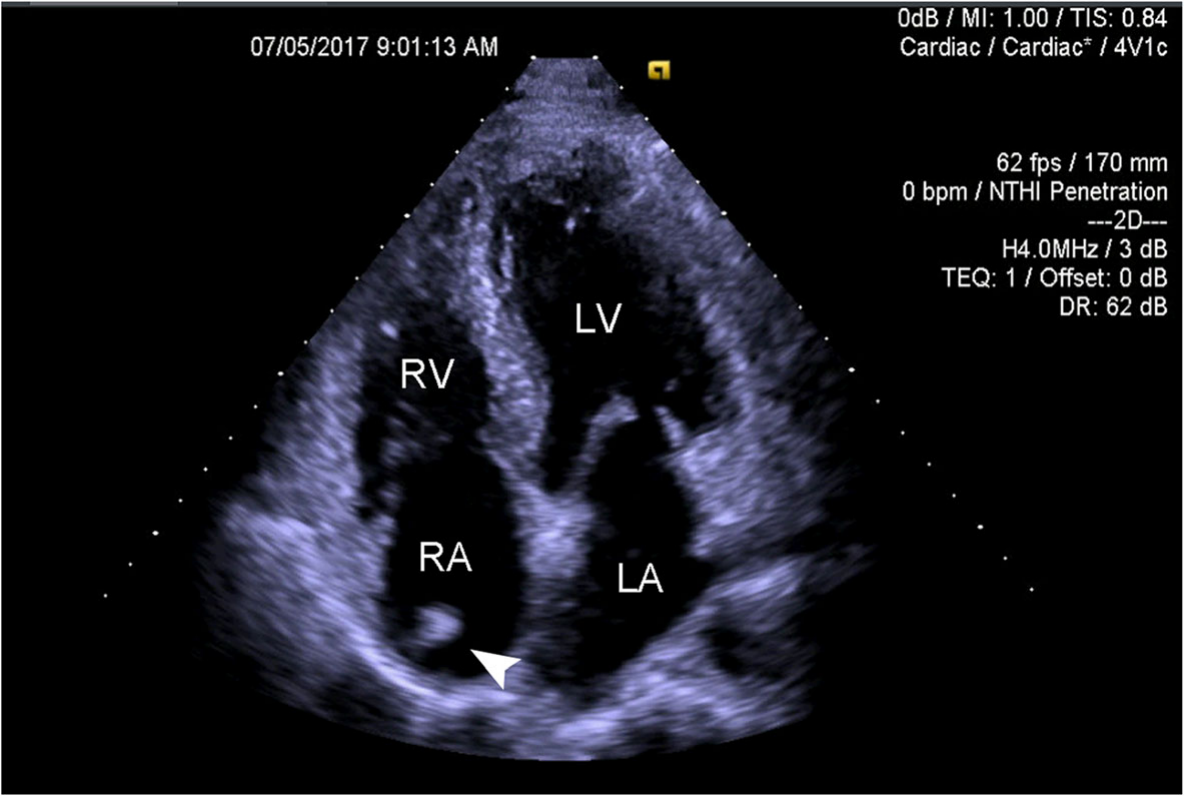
Transthoracic echocardiogram, in four-chamber apical view, showed an echogenic mass (arrowhead) in the right atrium. LA: left atrium, LV: left ventricle, RA: right atrium, RV: right ventricle
